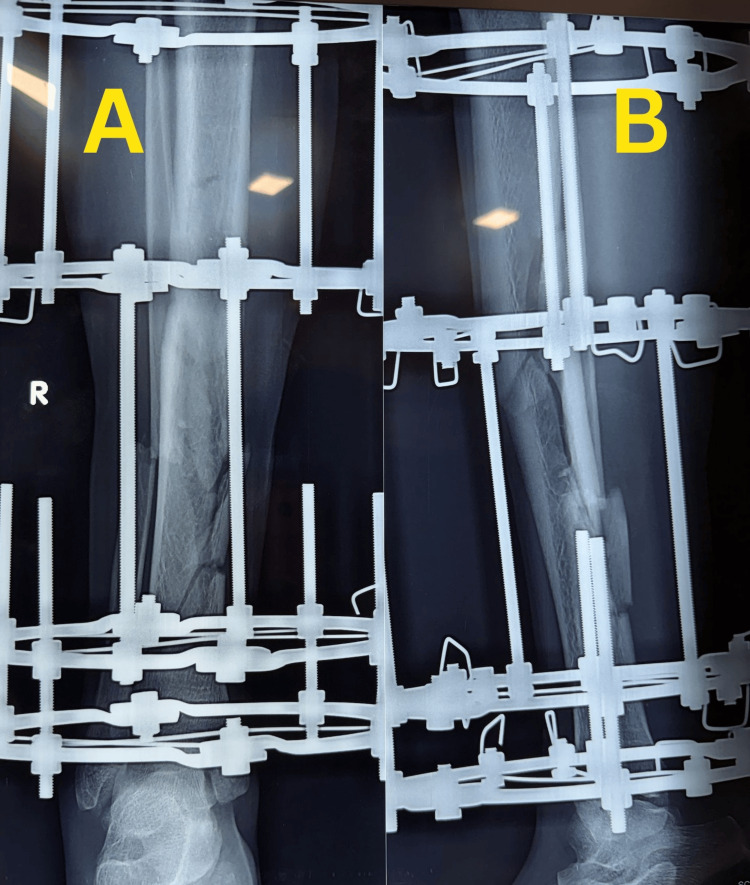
Follow-up radiographs of the right tibia Follow-up radiographs obtained on 23 December 2025 demonstrating maintained alignment of the tibial fracture with early callus formation: anteroposterior view (A) and lateral view (B).
